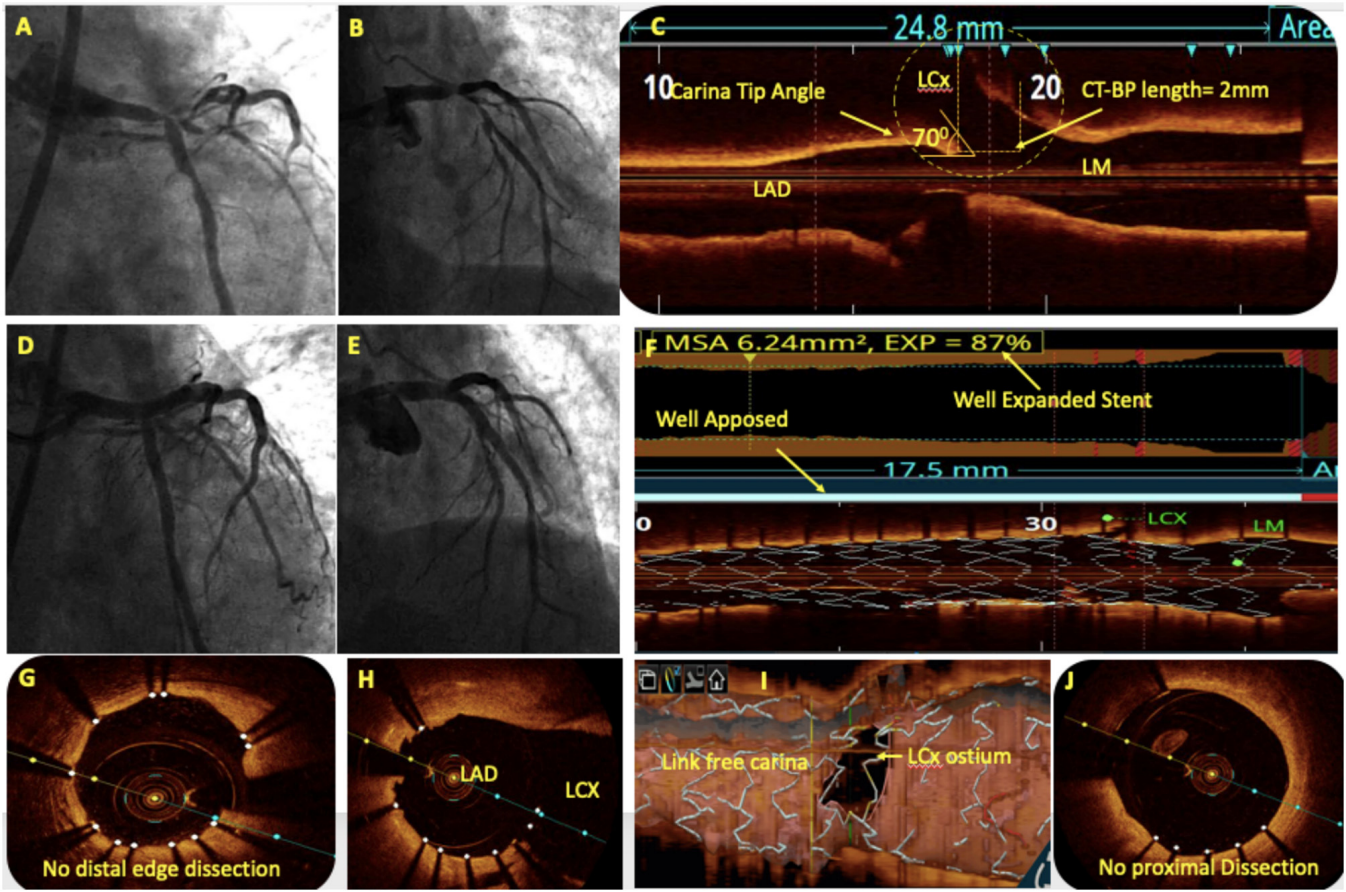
OCT in bifurcation angioplasty. (A,B) Coronary angiography showed a significant left main (LM) bifurcation lesion (Medina 1.1.1). (C) Pre-PCI OCT showed the carina tip (CT) angle of 70° and CT to bifurcation point (CT-BP length) of 2 mm suggestive of bifurcation lesion suitable for provisional stenting without the risk of side branch (SB) compromise. (D) An OCT-guided LM-LAD cross-over stenting was done followed by the proximal optimization technique (POT) with balloons of appropriate size. (E) Post-PCI angiography showed TIMI III flow in left coronary system without LCx compromise. Post-PCI OCT showed well-apposed stent with 87% expansion (F), without proximal or distal edge dissection (G,J) and minimal stent struts across LCx ostium (H). 3D reconstruction showed minimal inappropriate stent apposition across LCx ostium with link-free carina (I).
